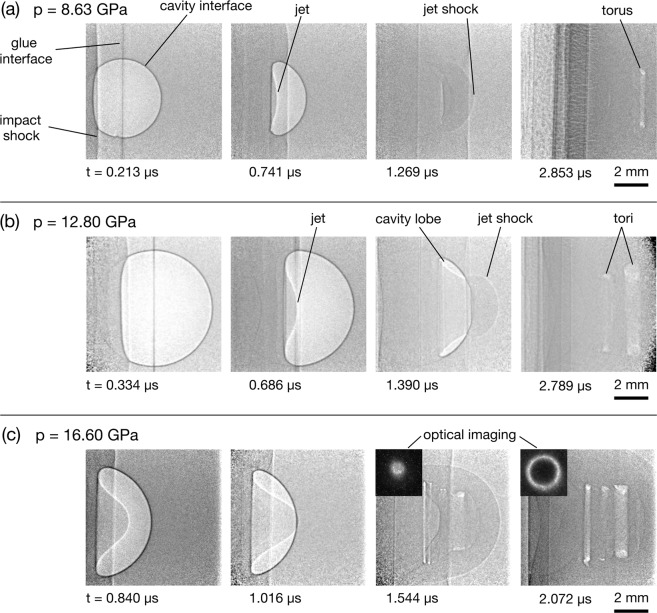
Radiograph sequences showing shock-cavity interactions in the hydrodynamic regime. (a) A 4 mm cavity and 8.63 GPa shock. (b) A 6 mm cavity and 12.80 GPa shock. (c) A 6 mm cavity and 16.60 GPa shock, with insets showing the rear-surface optical images of the toroidal plasma emission.
